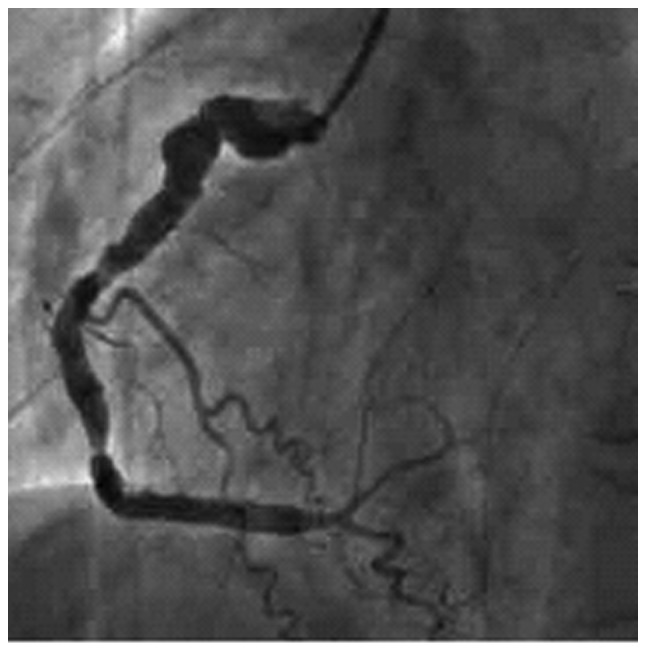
Stenosis was detected in the right coronary artery by selective X-ray coronary angiography. One is moderate stenosis in the proximal area and the other is severe stenosis in the distal area.
